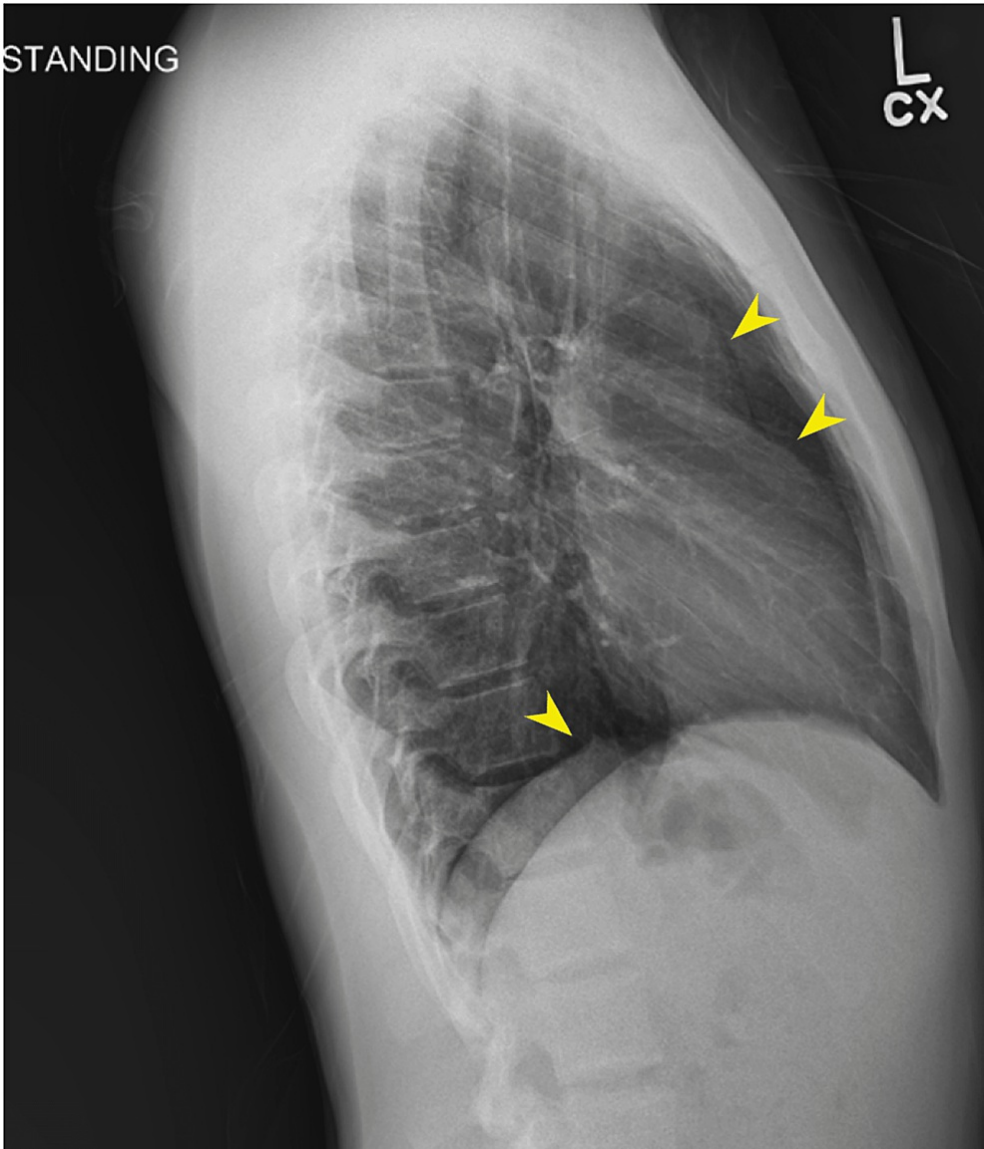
Lateral chest X-ray Yellow arrows: pneumomediastinum
